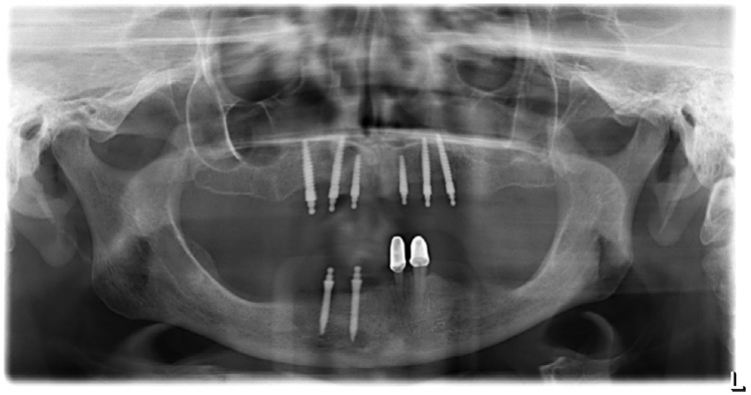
Panoramic radiography image with various mini-implants.
